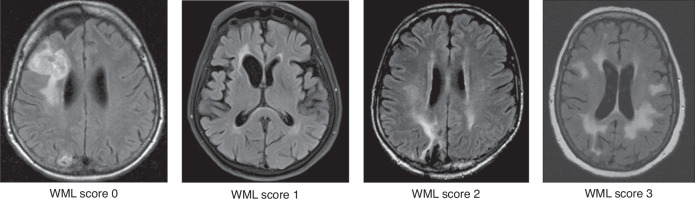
Exemplary MRI scans scored by the modified Fazekas score (mFS) from score 0 to 3 for the overall white matter lesion (WML) burden. All exemplary scans are from PCNSL patients. The assessment did not include specific lesions, such as tumor infiltration, post-therapeutic, or post-ischemic gliosis with surrounding edema.
